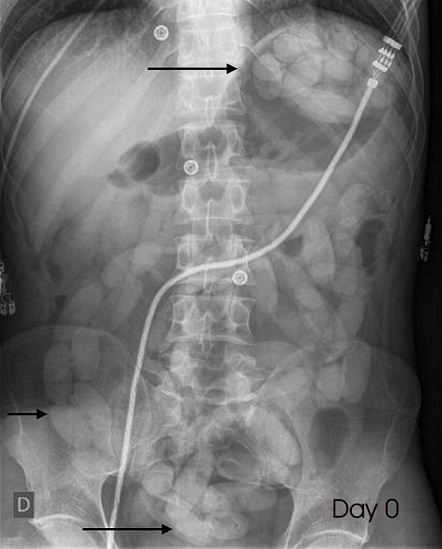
Plain abdominal X-ray at admission with multiple body packers (arrows)
